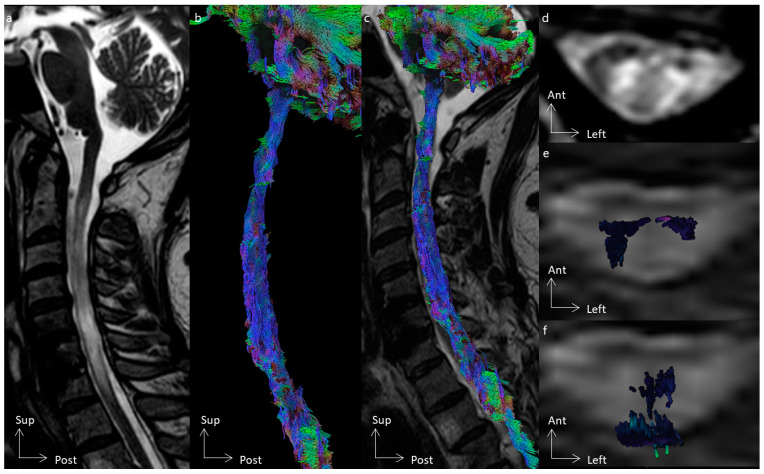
Spinal cord tractography in patient 1, with an astrocytoma. (a) Sagittal T2-weighted image showing a T2-weighted hypersignal at the C5–C6–C7 spine levels. (b) Sagittal view of the spinal cord tractography with 3D rendering. (c) Overlay of the spinal cord tractography on the sagittal T2-weighted MR images. (d) Axial T2-weighted images showing a diffuse T2-weighted hypersignal within the intramedullary tumor. (e) Overlay of the corticospinal tract on the axial T2-weighted MR images, within the tumor. (f) Overlay of the dorsal columns on the axial T2-weighted MR images, within the tumor and also compressed dorsally.
